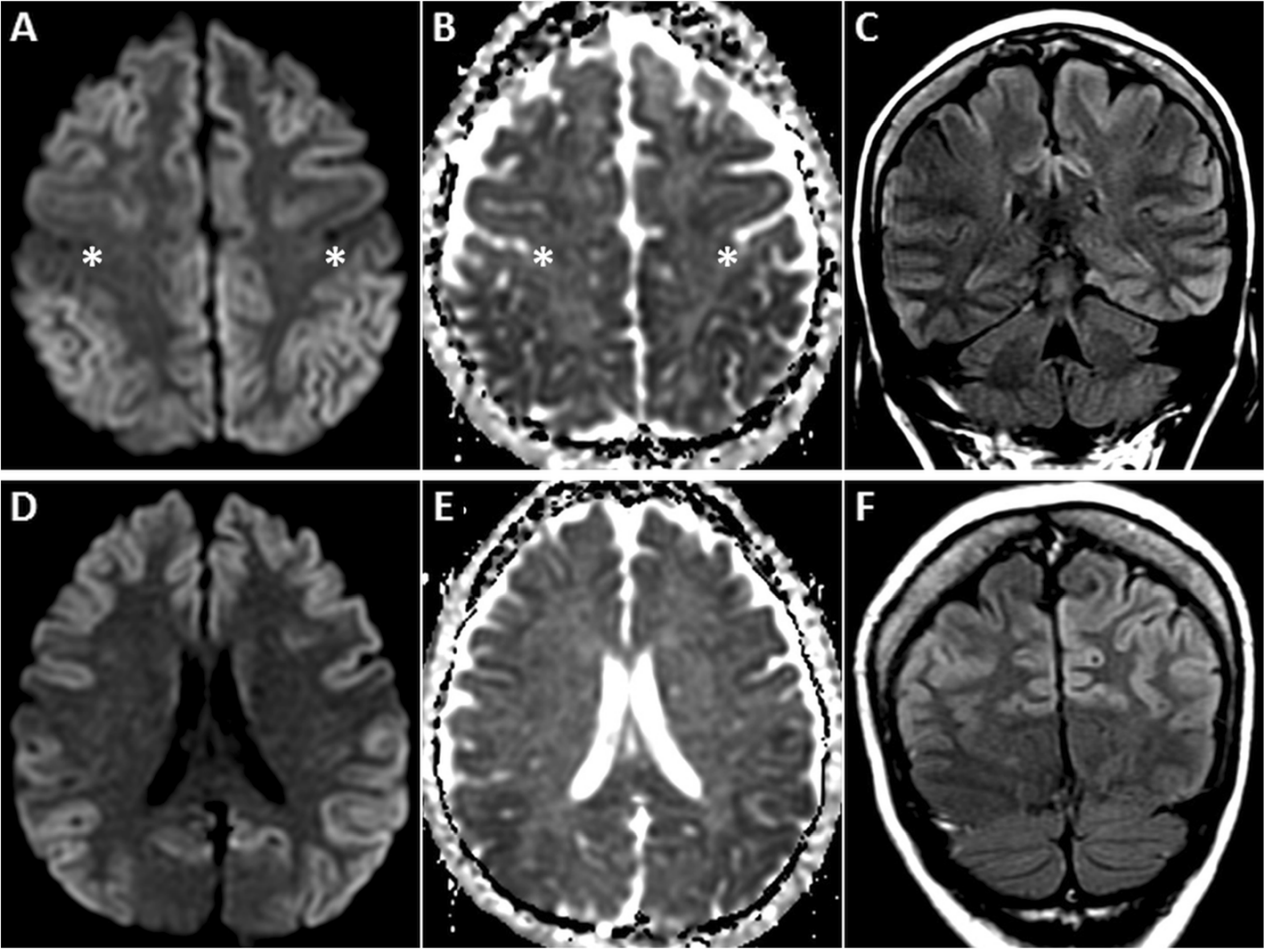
MRI of the brain in a 58-year-old female patient who presented with rapidly progressive dementia due to sCJD. Symmetric GRD is seen in the cerebral cortices on axial DWI (a, d) and ADC images (b, d, e) with sparing of the perirolandic regions (asterisks in a, b). Coronal FLAIR images (c, f) shows hyperintense signal in the affected cortices
